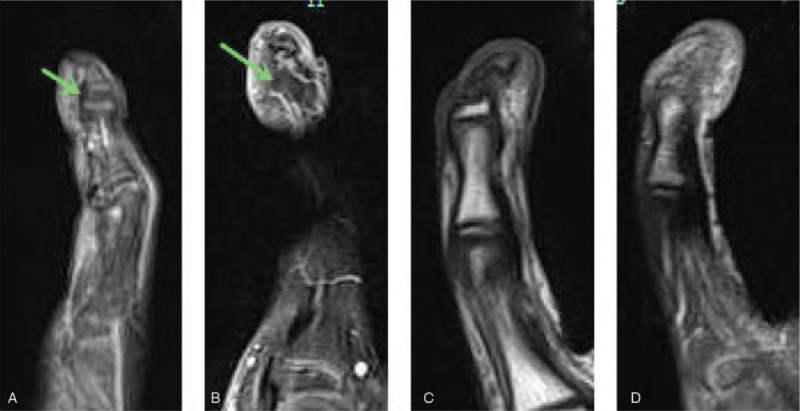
MRI of the right fifth finger, Normal level of tendon insertion (A). Wider and thicker growth plate of distal phalanx in the form of L-physis, but without any enhanced soft tissue (B–D).
